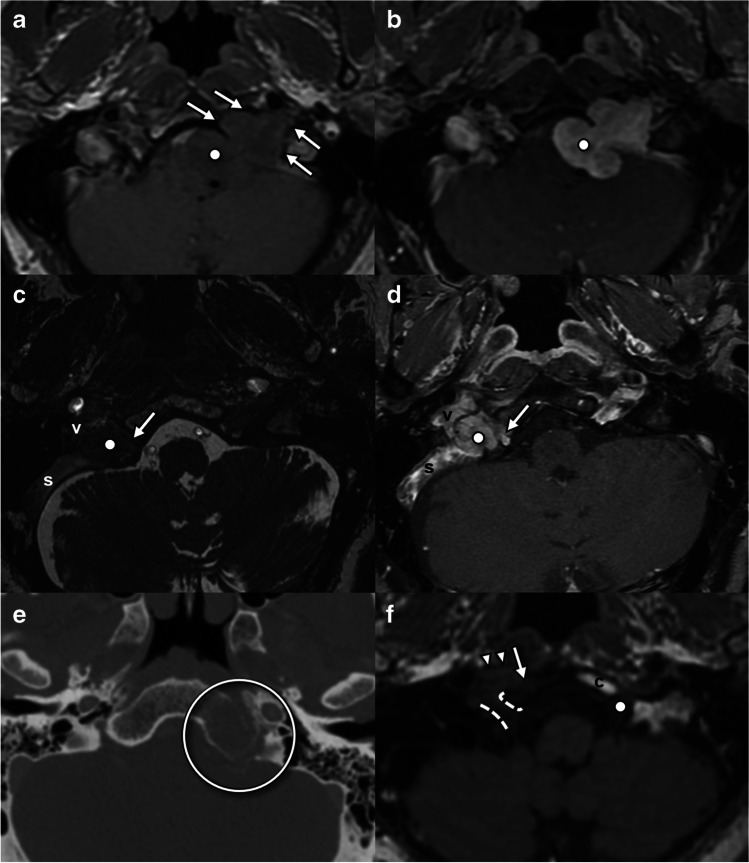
Lesions involving the intracanalicular segment of the hypoglossal nerve. a Axial T1W in a 44-year-old patient with chronic left-sided tongue weakness demonstrates a dumbbell-shaped mass (dot) extending from the left cerebellomedullary cistern causing smooth remodeling of the hypoglossal canal (arrows). b Axial post-gadolinium T1W in the same patient as a confirms marked, homogenous enhancement of the mass (dot), subsequently proven to be a schwannoma by fine needle aspiration. c Axial SSFP in a 55-year-old male with multiple right lower cranial neuropathies demonstrates a mass (dot) occupying the right jugular bulb, with involvement of the right hypoglossal nerve (arrow) in within the hypoglossal canal and with partial occlusion of the sigmoid sinus (s) and the internal jugular vein (v). d Axial post-gadolinium T1W in the same patient as c confirms enhancement of the mass, which was later biopsy proven to be a jugular paraganglioma. e Axial CT bone reconstructions following intravenous iodinated contrast in a patient with history of metastatic NSCLC demonstrates permeative erosion of the left basi-occiput involving the left hypoglossal canal (circle) due to a skull base metastasis. f Axial T1W in a 66-year-old patient with metastatic NSCLC demonstrates hypointense T1 signal in the right clivus and cortical destruction (arrow) due to a metastatic deposit with extraosseous extension (arrowheads). T1 hypointense mass involves the right hypoglossal canal (dashed lines). The normal left hypoglossal canal (dot) is hyperintense on T1W and surrounded by T1 hyperintense clival fatty marrow (c)
